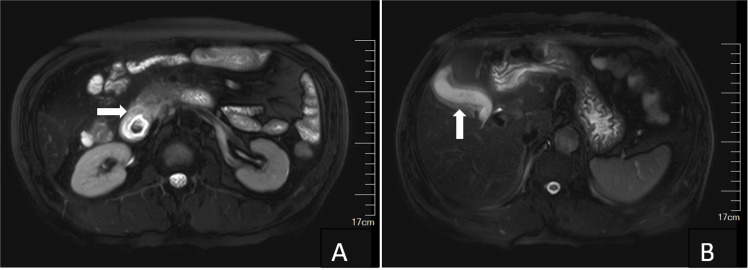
Axial-unenhanced MRI of the patient’s abdomen. T2-weighted image demonstrated slight effusion around the head of the pancreas and thickening of the descending part of the duodenum (A, white arrow), signs of chronic cholecystitis, and sludge-like gallstones (B, white arrow). MRI: magnetic resonance imaging
